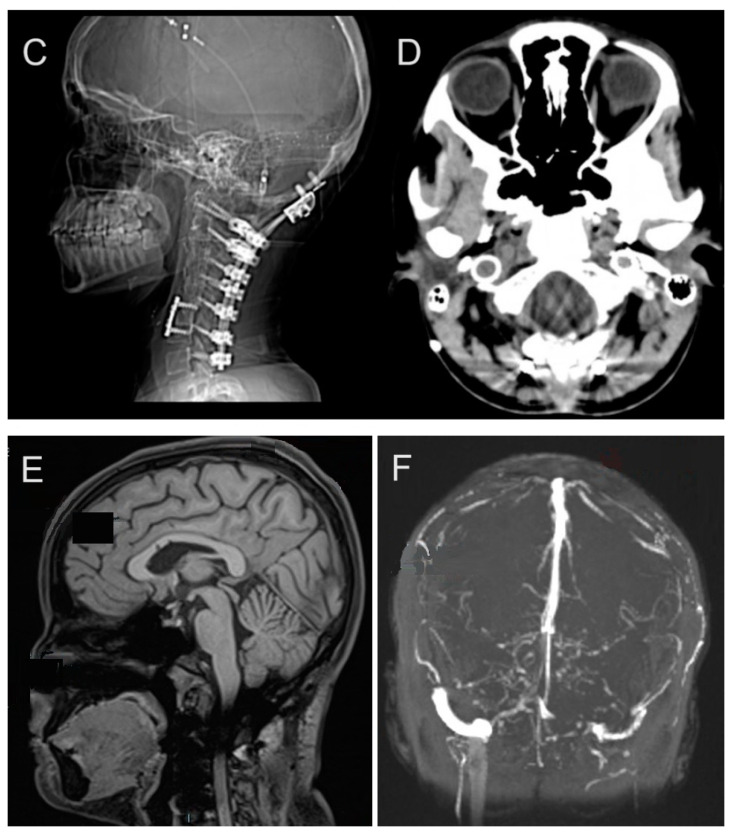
Longitudinal imaging findings in a patient with hEDS demonstrate progressive cervical spine instability and vascular complications. (A,B) A 2015 CT scan at age 15 showing post-surgical changes including occipital-C2 fusion and C2–C5 ACDF, with cervical lordosis straightening and C5–C6 anterolisthesis. Prior interventions included tethered cord release at age 12 and lumbar shunt placement at age 14 for IIH management. (C,D) CT in 2019 revealing expanded occipital-to-C7 fusion after hardware removal, necessitated by progressive cervical instability. (E) Brain MRI in 2016 demonstrates normal intracranial findings without restricted diffusion, hemorrhage, or tonsillar ectopia. (F) A 2016 angiogram showing asymmetric jugular veins with right-sided dominance, post-jugular foramen stenosis, and diminished left-sided flow.
